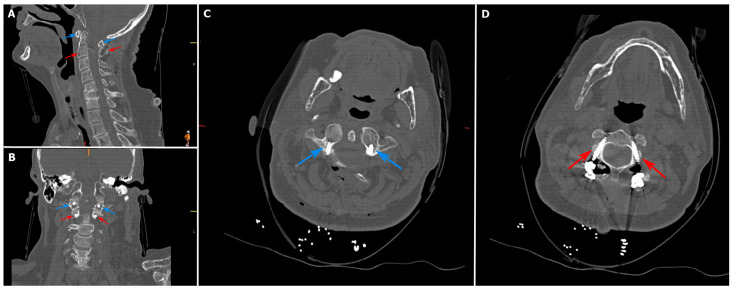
Grade A Gertzbein and Robbins screws applied in the study cohort. (A): Corrected atlantodental interval on sagittal computed tomography (CT); (B): screw positioning on coronal CT; (C): placement of C1 screws; (D): placement of C2 screws. Blue arrow: C1 vertebra and screws; Red arrow: C2 vertebra and screws.
